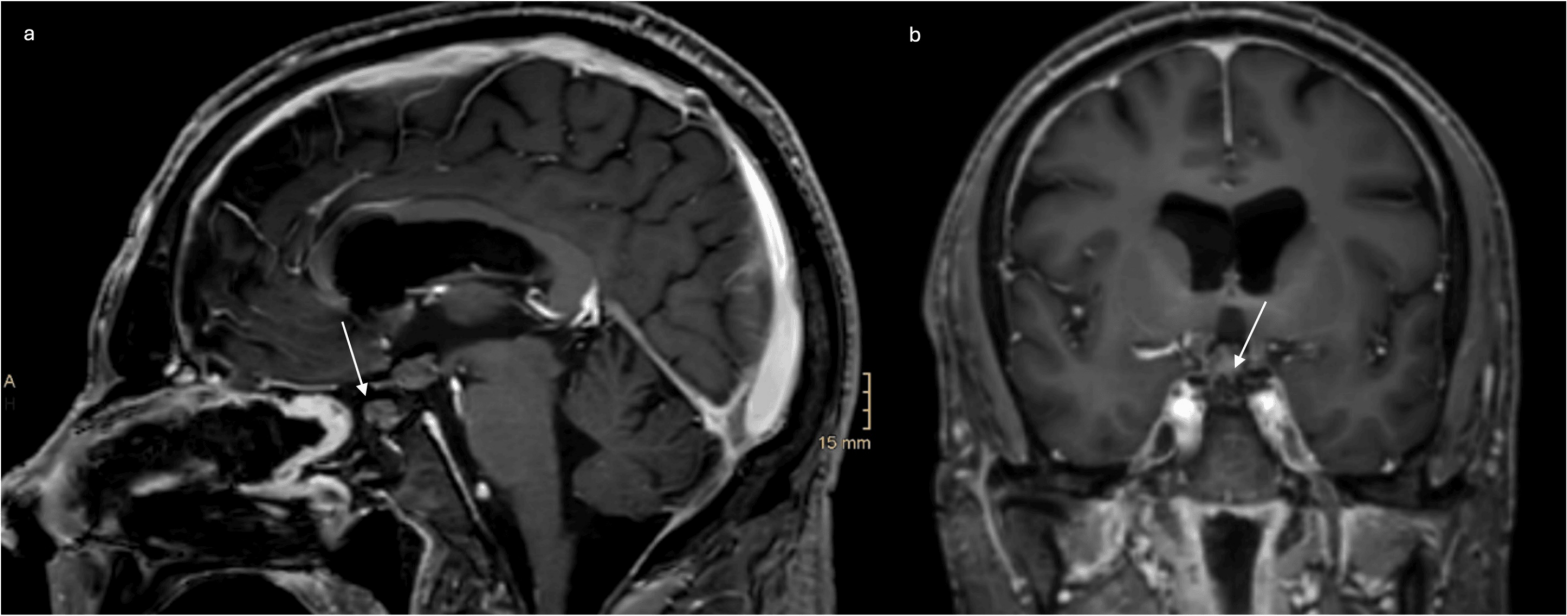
Postoperative cranial MRI with contrast medium Postoperative (a) sagittal and (b) coronal T1-weighted MRI showing complete removal of the metastasis. The arrows point at the abdominal fat graft that was used for the skull base reconstruction.
